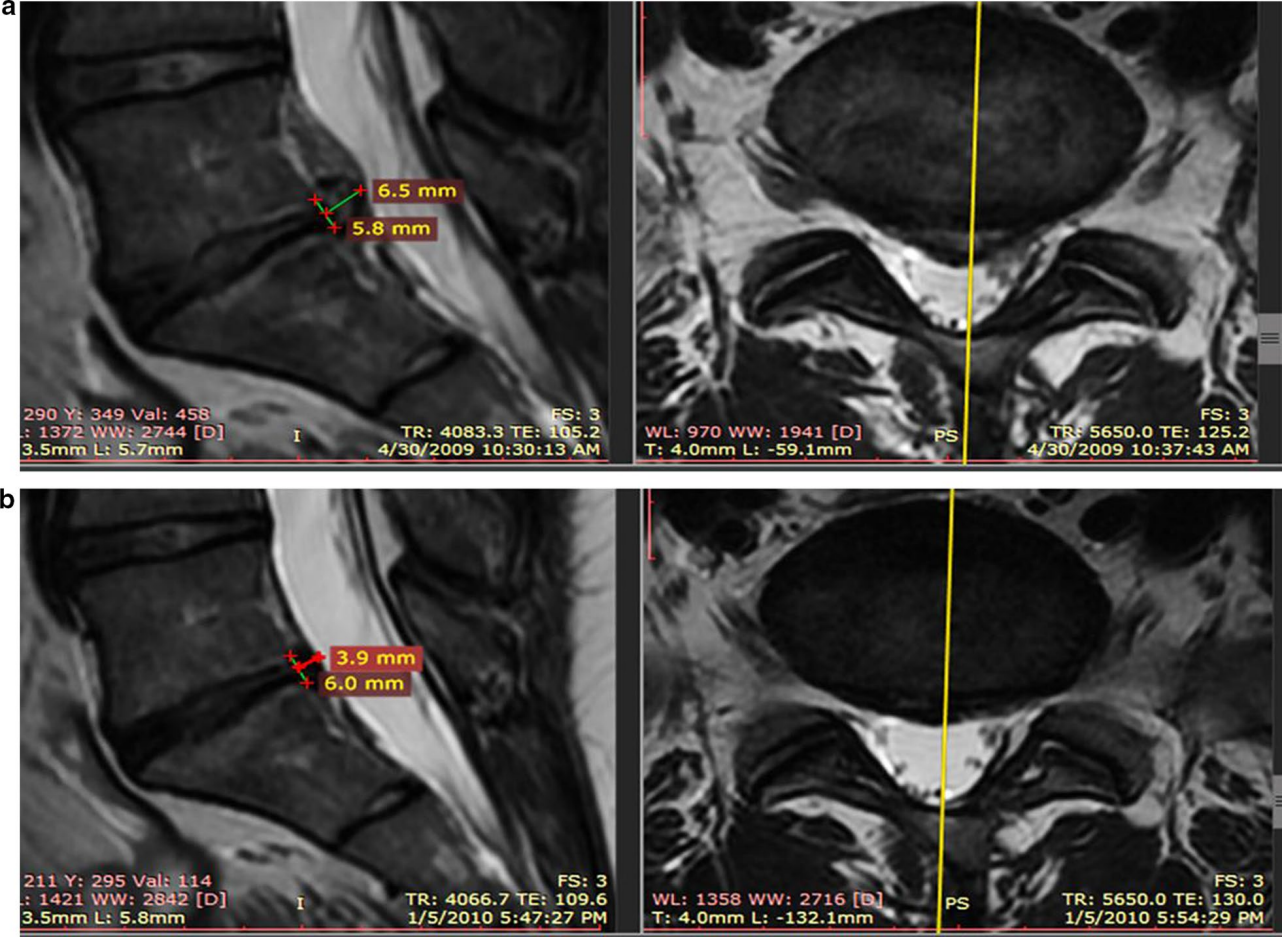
Comparison of disc bulge size on MRI. a Pre-treatment MRI, L5-S1, 4-30-2009; 6.5 mm posterior disc bulge. b 5 months post-treatment MRI, L5-S1, 1-5-2010; 3.9 mm posterior disc bulge
